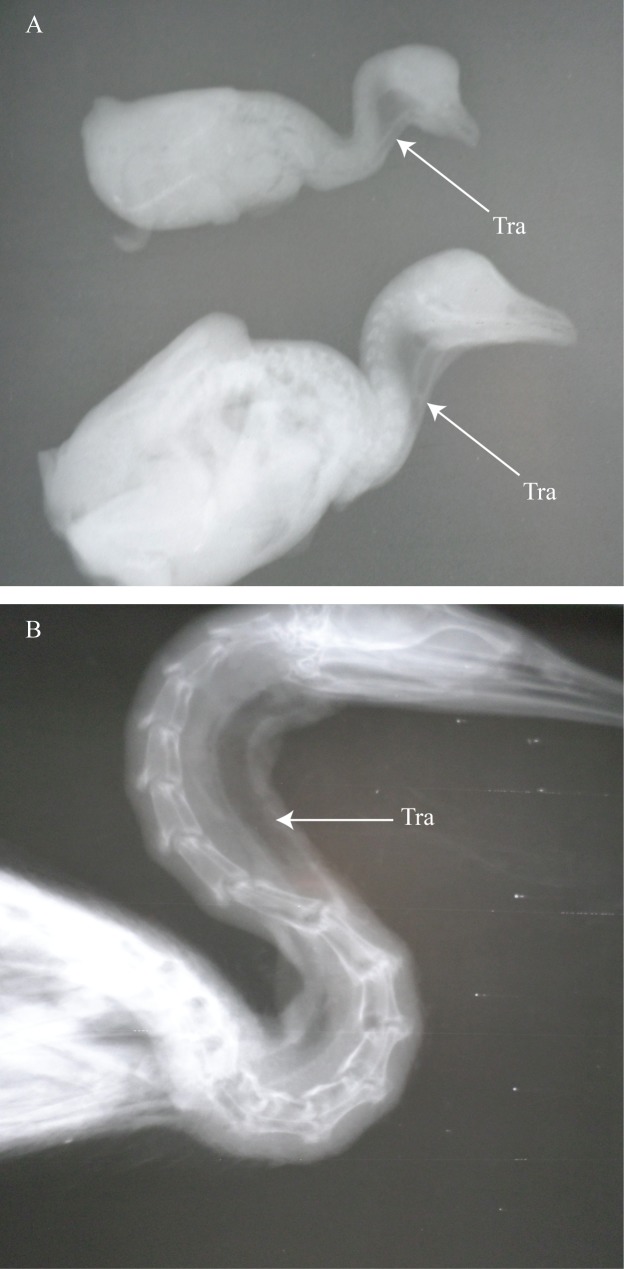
Phalacrocorax auritus* radiographs. (A) hatchling double-crested cormorants. (B) adult. Tra = trachea.
